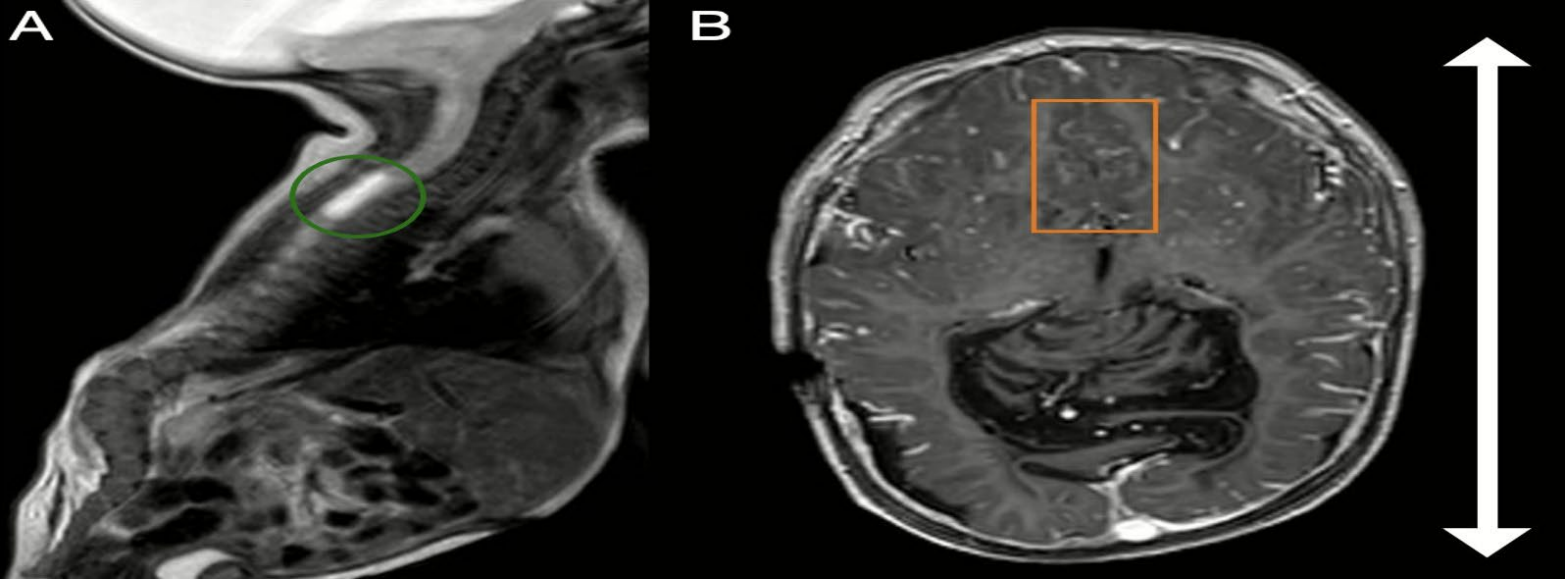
(A) Selected sagittal T2‐weighted MRI image of the spine demonstrates a dilated central canal extending from approximately D1 to D3 vertebral levels, consistent with a small syrinx (green circle). (B) Selected axial contrast‐enhanced T1‐weighted MRI image of the brain demonstrates a fenestrated falx cerebri, manifested by interdigitating gyri within the midline defect (orange rectangle). Note the increased anteroposterior (AP) diameter of the skull, indicated by a bidirectional double‐headed white arrow, suggesting scaphocephaly.
